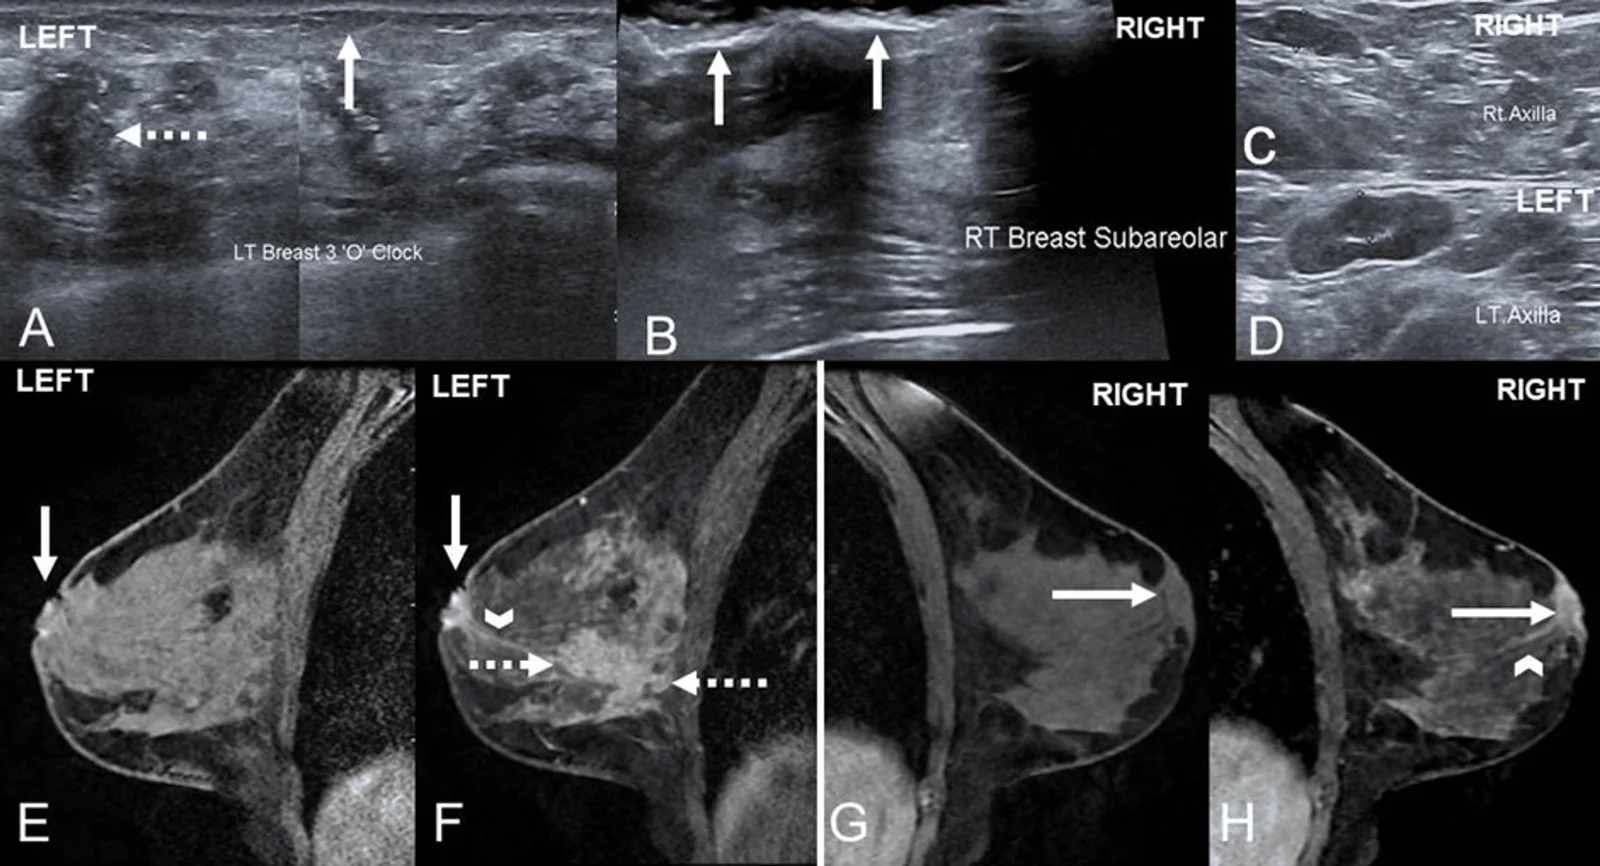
A case of bilateral Paget's disease of the breast CEMR: contrast-enhanced magnetic resonance (imaging) (A-B) Ultrasonogram demonstrating bilateral skin thickening involving the nipple-areola complex (solid arrows), irregular hypoechoic nonmass area with calcific specks (dotted arrow), and (C-D) bilateral axillary lymphadenopathy. (E-F) CEMR demonstrates nipple erosion (solid arrow), regional nonmass enhancement (dotted arrows) in the lower outer quadrant of the left breast extending to the eroded nipple (arrowhead). (G-H) Intensely enhancing nipple areolar complex (solid arrow), along with linear nonmass enhancement, right breast (arrowhead)
